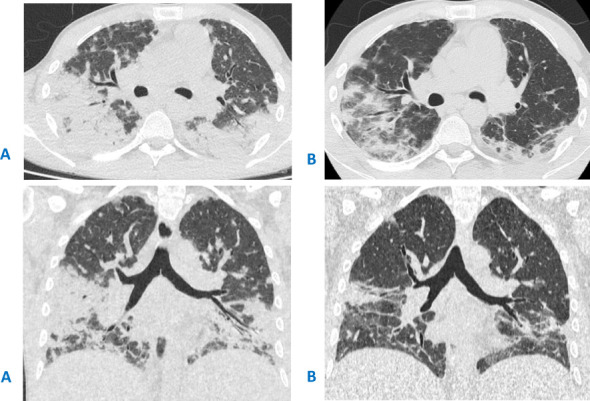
(A) CT scan before the thoracoscopic lung biopsy and steroid initiation, showing massive bilateral areas of airless lung tissue, more prominent in the lower lobes, located in both central and peripheral areas. (B) CT scan after 2 weeks of treatment with high doses of steroids.
